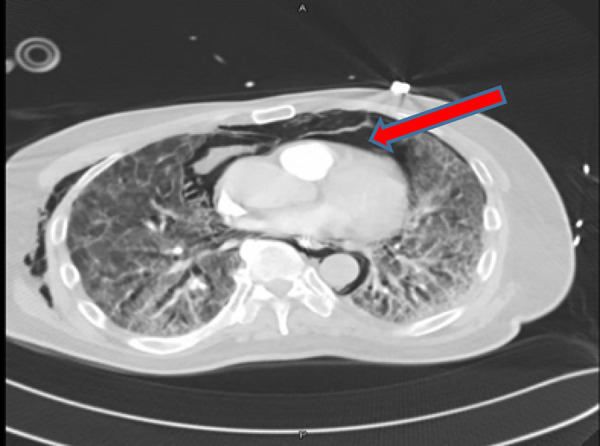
Computed tomography scan of the chest showing tension pneumomediastinum
